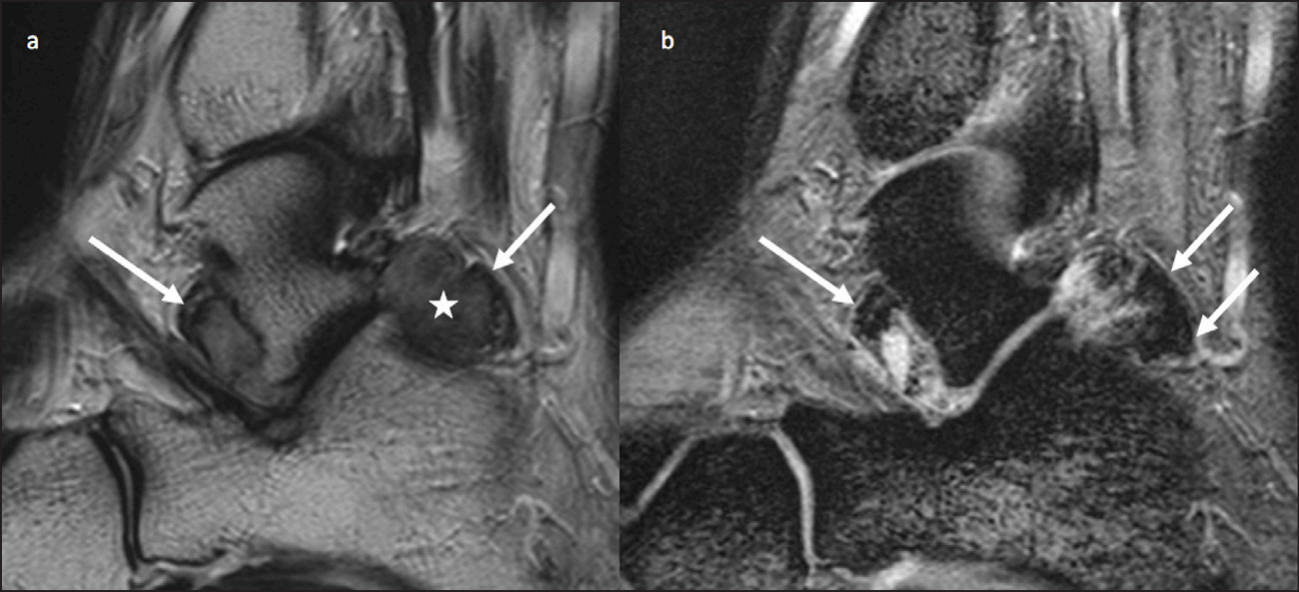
a. Sagittal Turbo Spin-Echo T2-weighted image shows an hypointense mass (white star) with low signal intensity foci corresponding to hemosiderin deposition (white arrows). b. On Sagittal Gradient Echo T2-weighted images, hemosiderin deposition appears more visible because of the blooming effect due to the magnetic susceptibility (white arrows).
